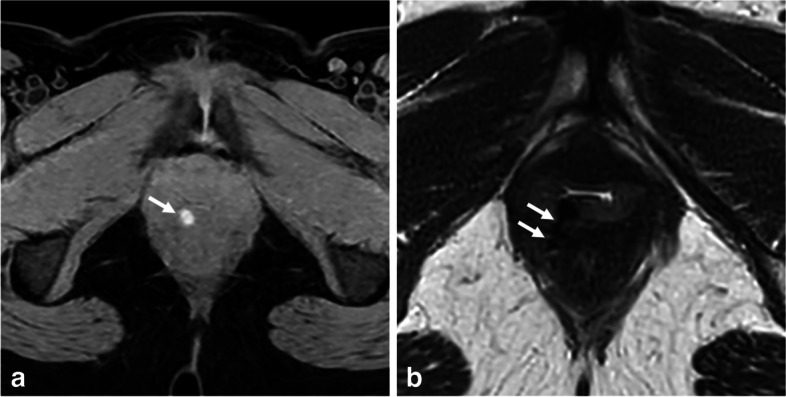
Vaginal epithelial inclusion cyst in a 35-year-old woman with non-cyclic perineal pain and palpable vaginal induration, 1 year after childbirth with episiotomy. a Axial T1-W fat-suppressed MR image shows a single small T1-hyperintense cyst (arrow) in the posterior vaginal wall. b Axial T2-W MR image shows a T2-hypointense cyst and T2-hypointense thin linear fibrous scar tissue within the puborectalis muscle (arrows), consistent with an episiotomy scar
